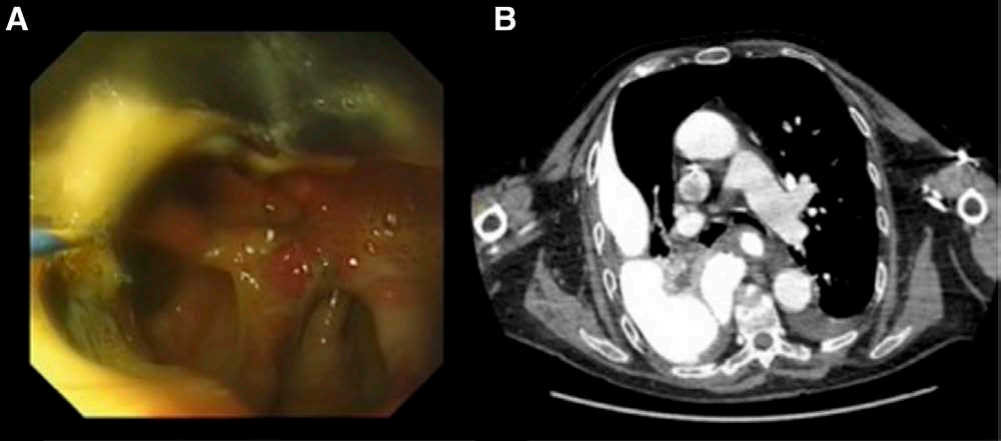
An endoscopic image (A) and a cross-sectional CT image (B) of an anastomotic leak. CT = computed tomography.
